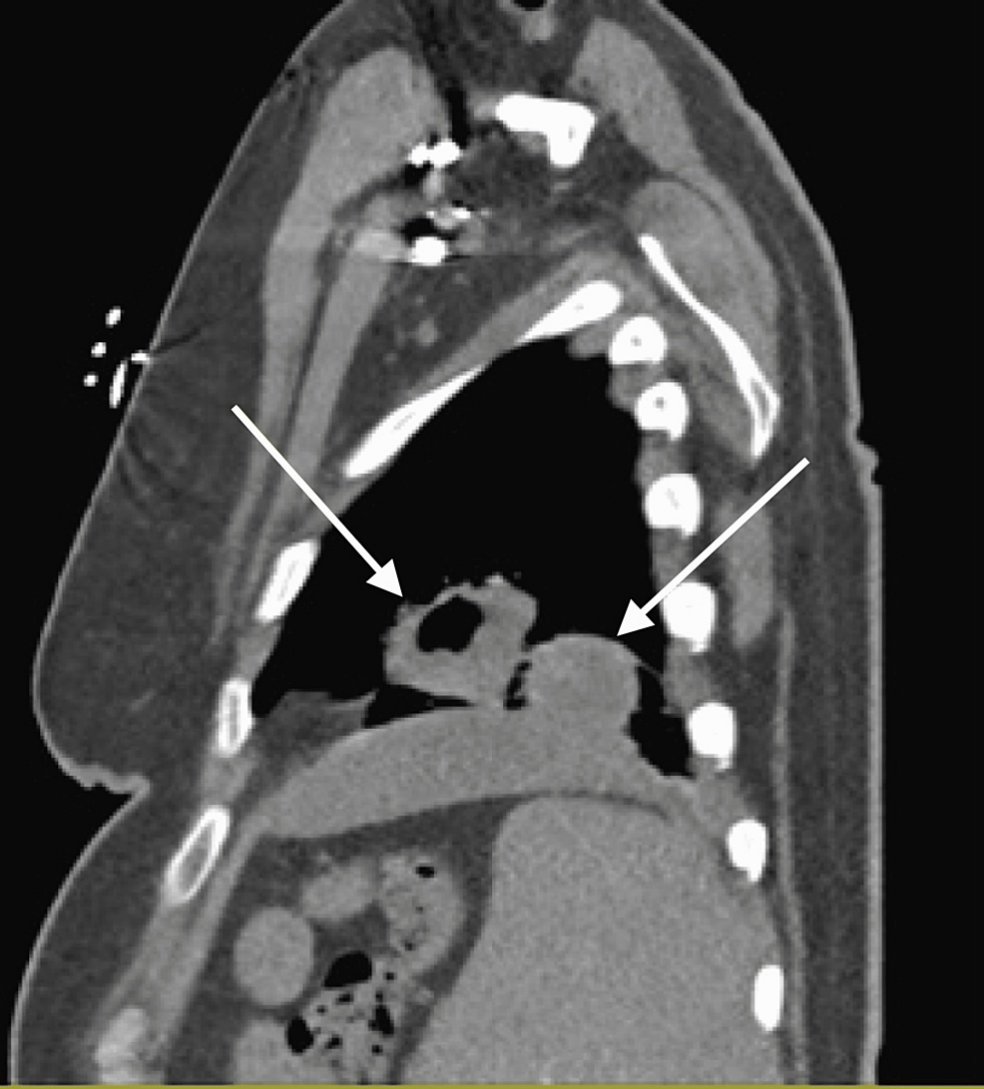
Chest CT (sagittal view) demonstrating two left lower lobe cavitary lesions.
